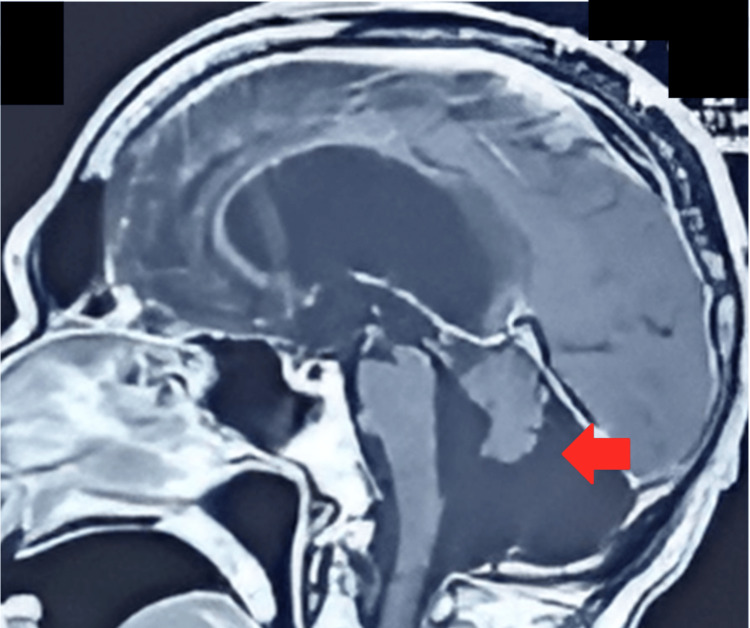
Brain MRI without contrast sagittal view showing a hypoplastic cerebellum and a CSF signal intensity area (arrow) in the posterior fossa communicating with the enlarged fourth ventricle anteriorly
